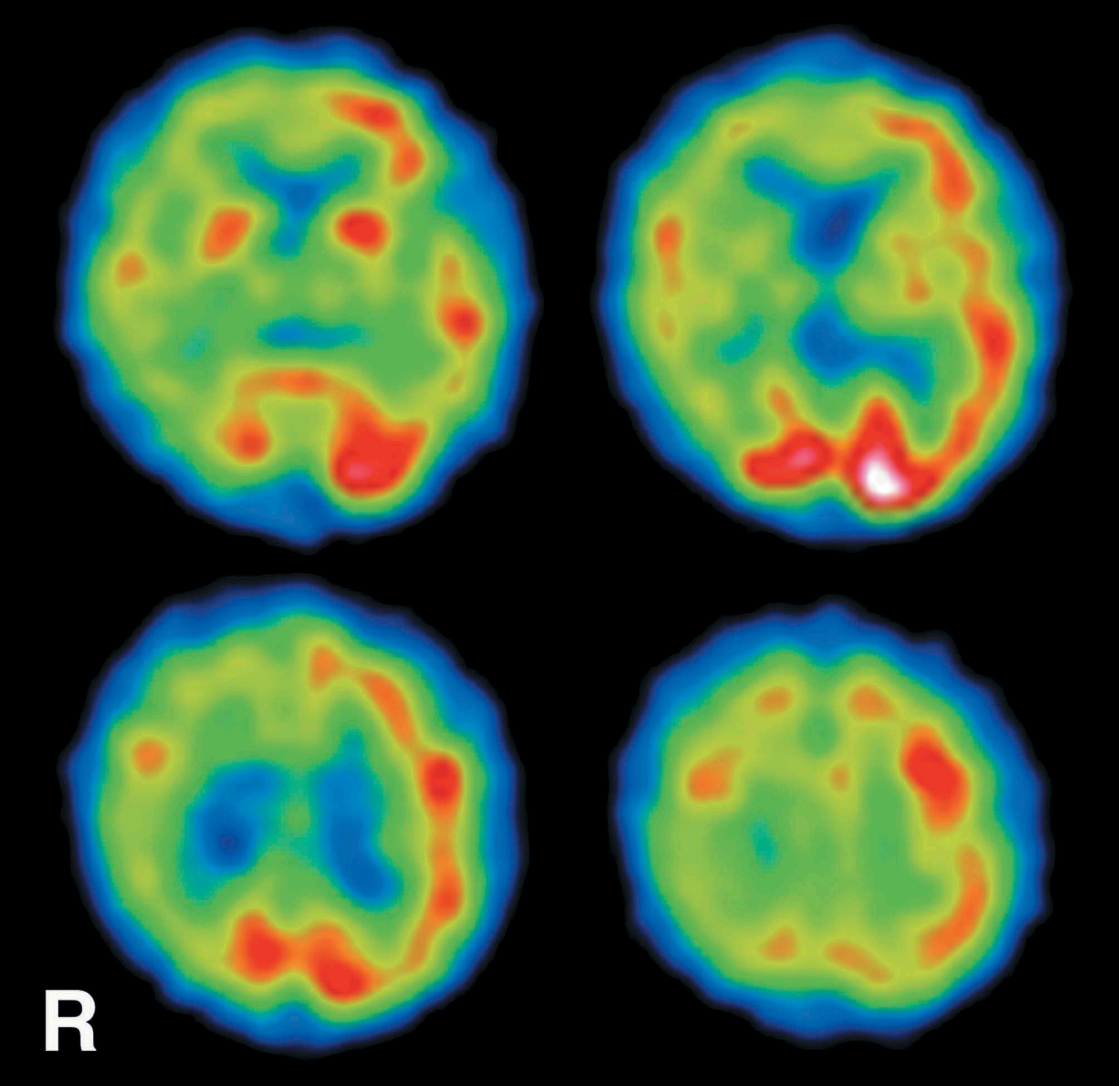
SPECT findings. ^99m^Tc-ECD-SPECT images obtained 5 months after the onset of symptoms revealed decreased regional cerebral blood flow in the right cerebral hemisphere, particularly in the parietal lobe. The regions with decreased cerebral blood flow almost corresponded to the regions that were hyperintense on diffusion weighted magnetic resonance imaging. ^99m^Tc-ECD-SPECT, technetium-99m ethyl cysteinate dimer single photon emission computed tomography; R, right side.
